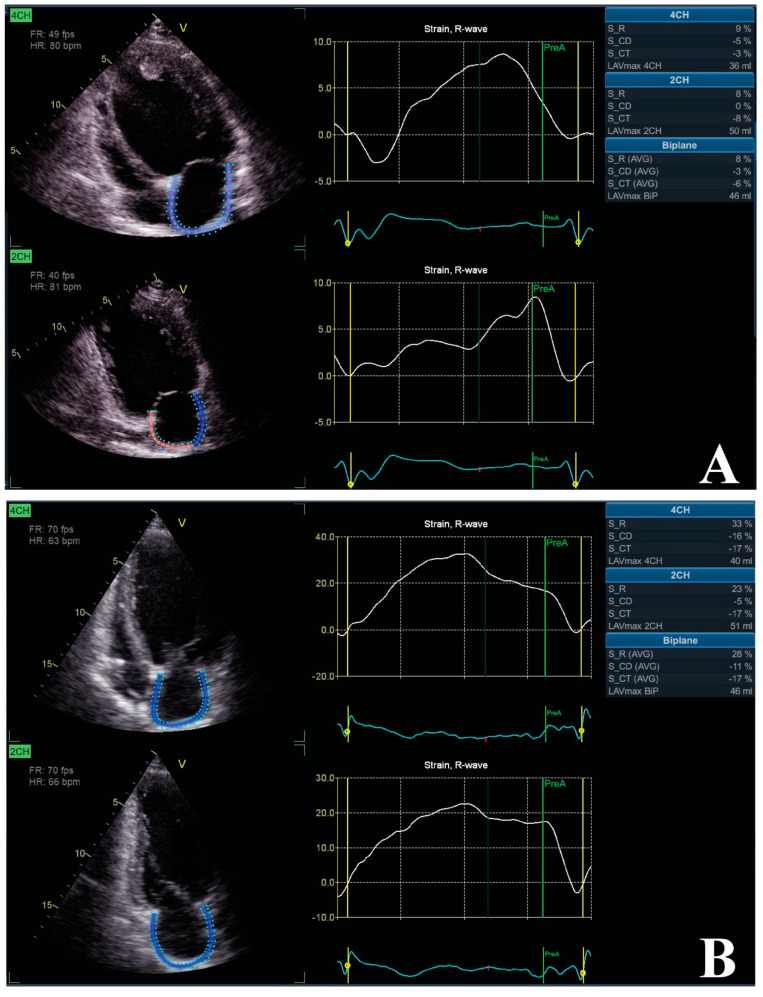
The impact of CRT on LA strain. Patient with dilated cardiomyopathy, LVEF of 33%, and QRS morphology of LBBB with duration of 153 msec. Echocardiographic evaluation using a General Electric VIVID E95 vendor and EchoPac software package to determine LA strains (Images from the personal collection of the authors). Initial evaluation showed impaired LA strains (A) and, after a 6-month follow-up, there is significant LA functional reverse remodeling with improved LA strains (B). Abbreviations: LA, left atrium; LBBB, left bundle branch block; LVEF, left ventricle ejection fraction.
